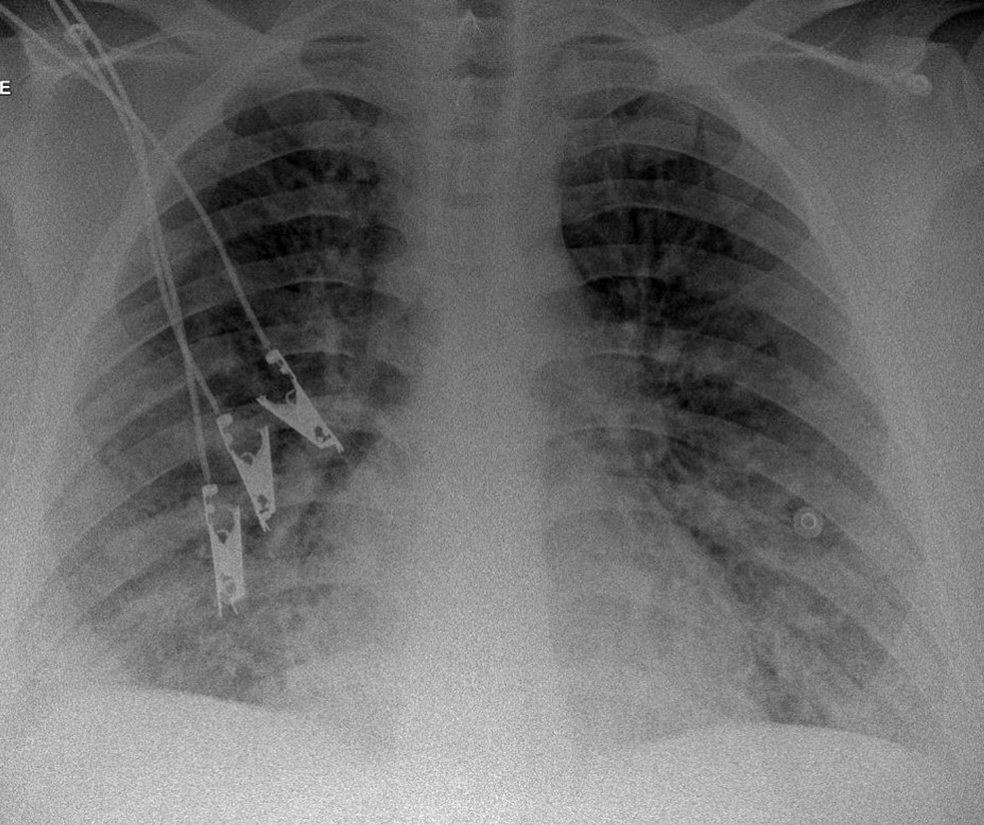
Chest X-ray Bilateral fluffy alveolar infiltrates predominantly affecting lower zones with blunting of both costophrenic angles.
